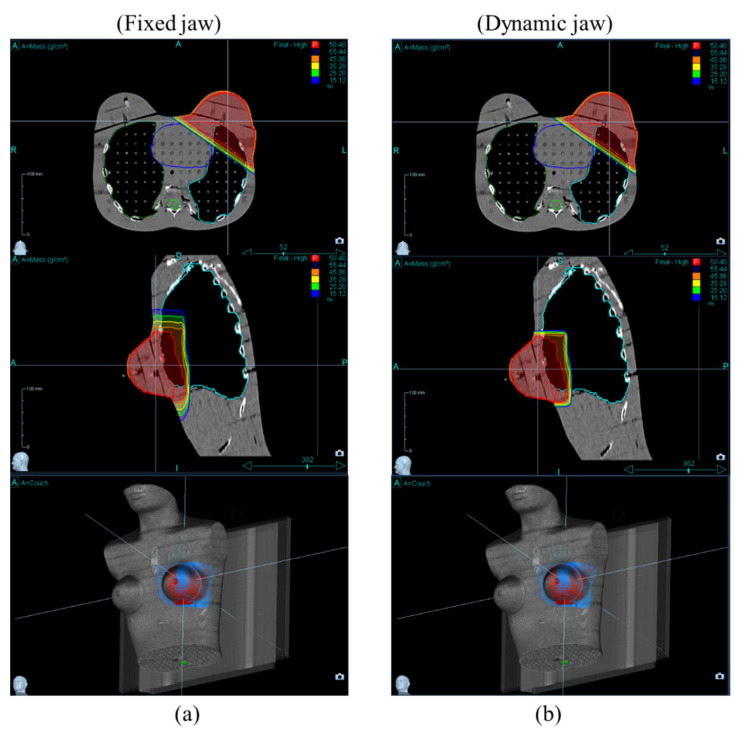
CT images (upper and middle rows) and 3D dose distribution (lower row) between jaw mode at (a) fixed and (b) dynamic jaw modes at the 5.0 cm field width. Red and blue colors indicate target coverage of more than 95% and 30%, respectively, of the prescription dose.
